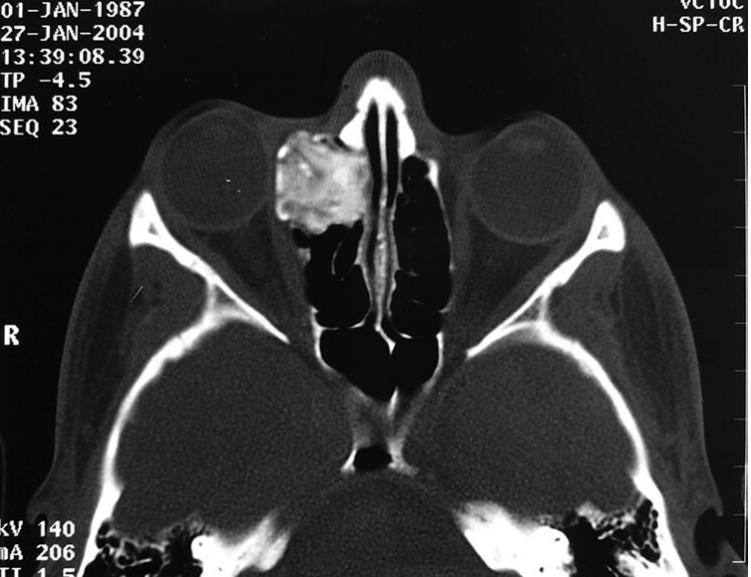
Preoperative CT scan. CT scan of the paranasalsinuses, demonstrating a bone density mass within the right ethmoidair cells, with well defined margins, extending laterally through thelamina papyracea, to the ipsilateral right orbit.
